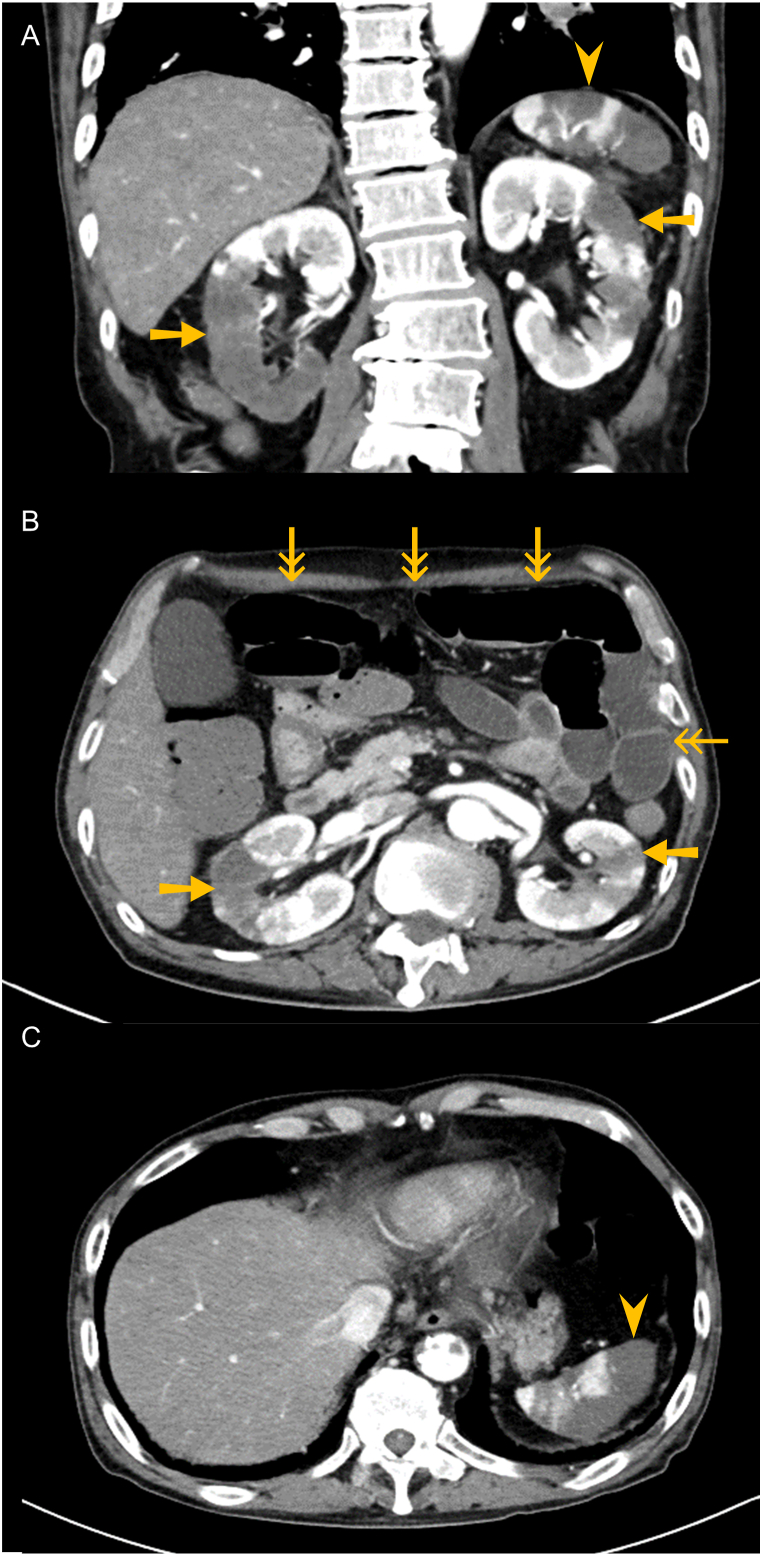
Enhanced abdominal computed tomography images. Enhanced abdominal computed tomography revealed bilateral kidney infarction (arrows), splenic infarction (arrowheads), and intestinal enlargement due to ileus (double arrows) on admission. Coronal (A) and horizontal (B, C) images.
